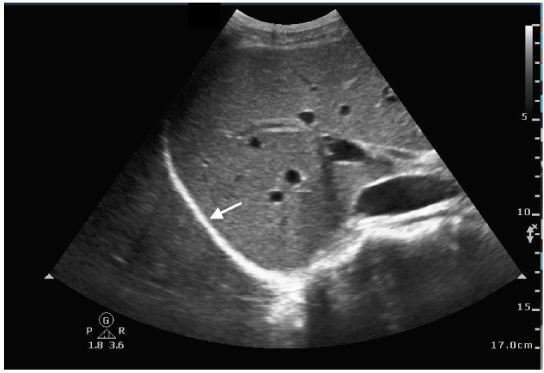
Ultrasound of normal lung: note the mirror image artifact: appearance of liver above and below the diaphragm (arrow) and the absence of a spine sign.
